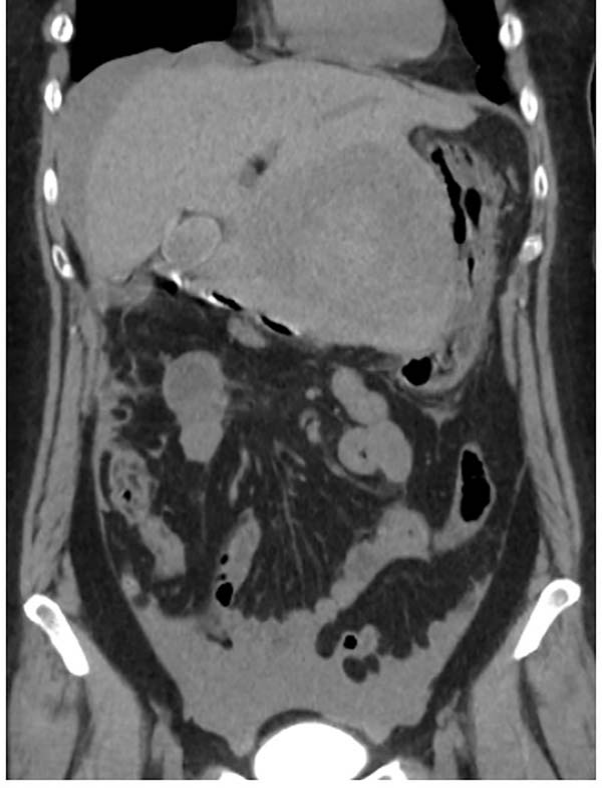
Abdominal CT at the onset of symptoms suggestive of acute abdomen. A 10 cm diameter liver tumor was seen to protrude from the left lateral segment of the liver and high-density ascites was identified around the liver.
